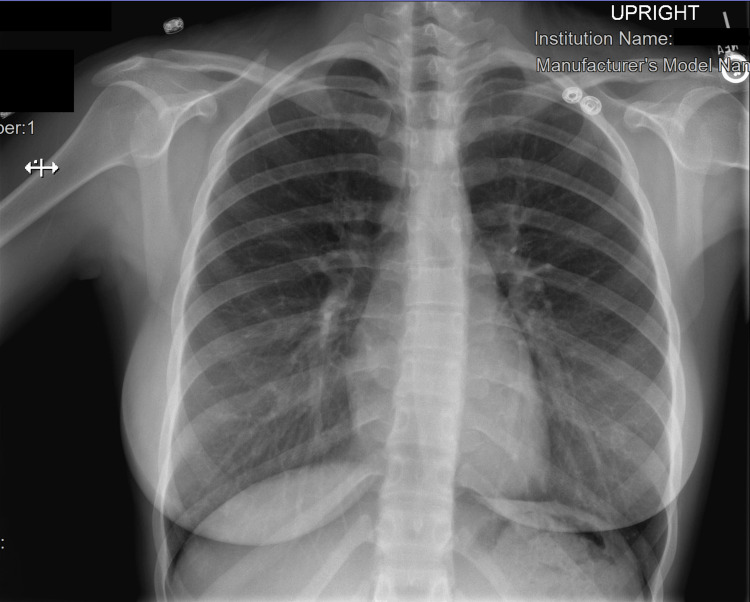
Chest x-ray of the patient showing mild levoscoliosis but no acute cardiopulmonary processes
